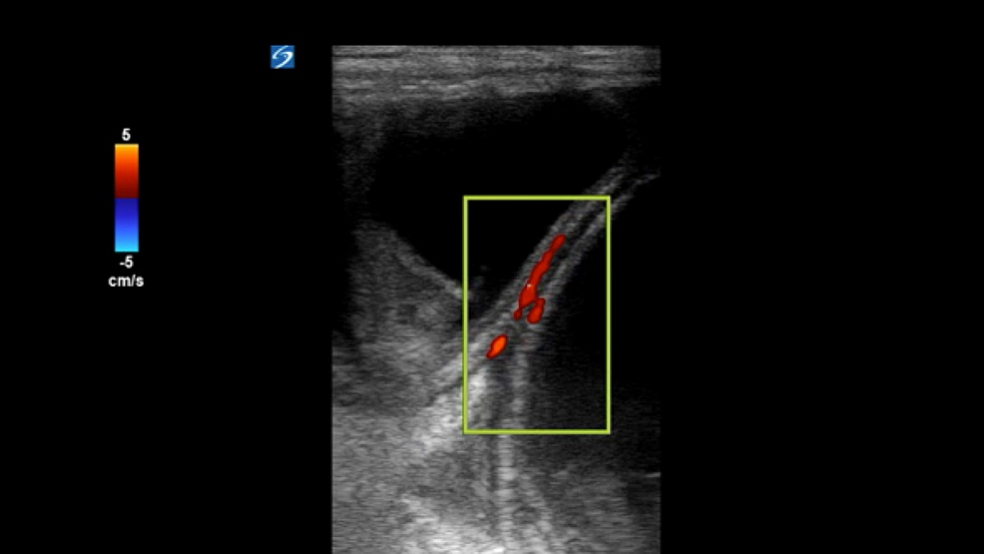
Preserved vascular flow in the bowel wall is identified via Doppler imaging.
